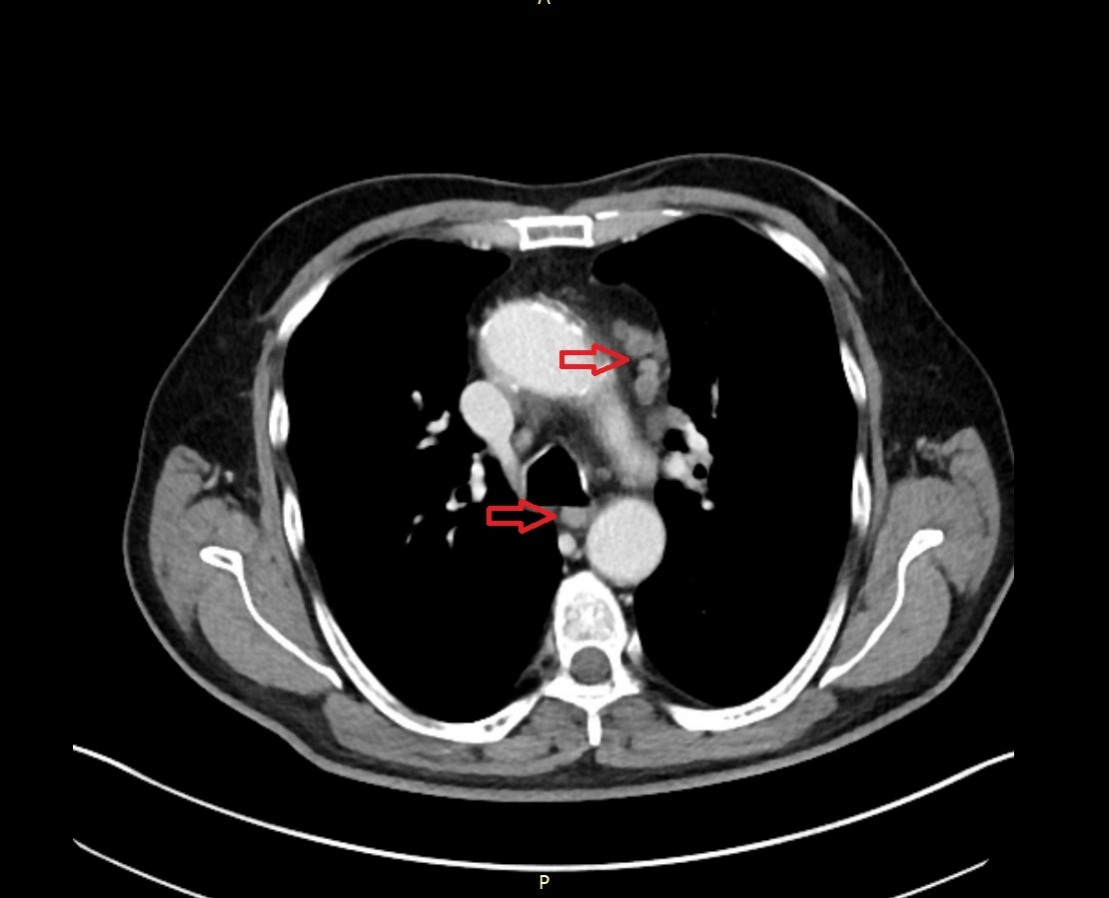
CT scan. The image shows suspect mediastinal lymph nodes. CT: computed tomography
